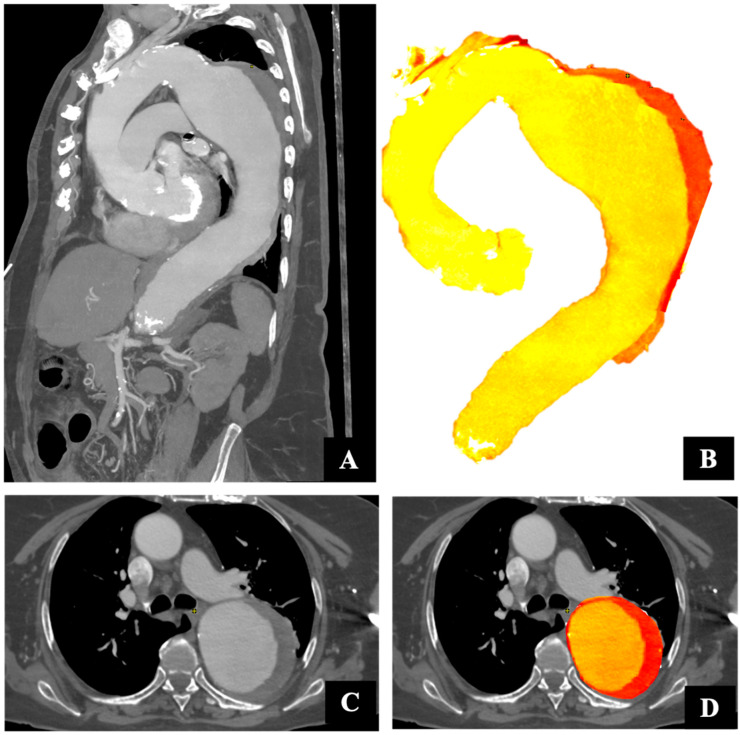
Thoracic aortic volume. Preoperative sagittal (A,B) and axial (C,D) computed tomography images. The yellow area indicates the aortic lumen, and the red area indicates the thrombus and wall.
